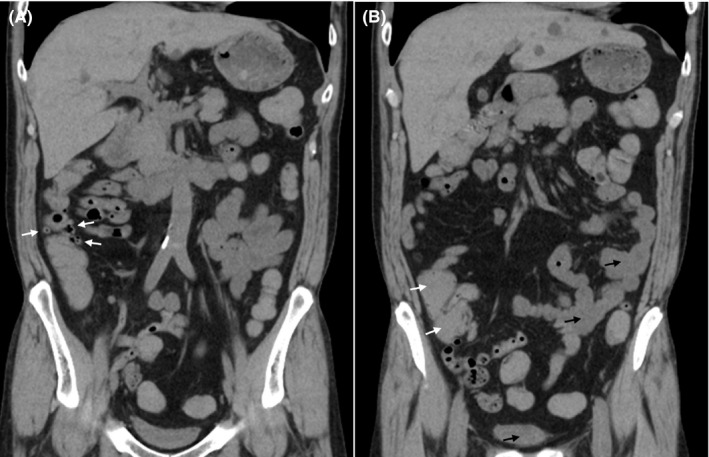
A, Nonenhanced computed tomography showed the presence of diverticula (white arrow), as gas‐filled outpouchings from the ascending colon. B, CT revealed high‐density contents in right colon (white arrow) compared with the small bowel and rectum (black arrow): the CT values in the cecum were more than twice the small intestine and rectum CT values
